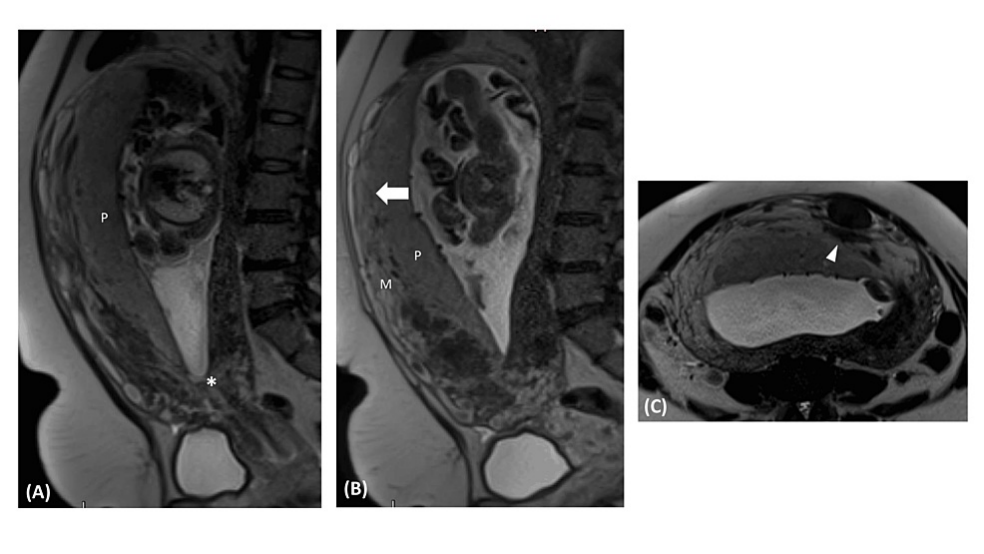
Fetal MRI. (A) Sagittal T2 single-shot fast spin echo (SSFSE) shows anterior placenta (P) in an abnormally low-lying position, distancing 1.4 cm from internal cervical os (*), diagnosing placenta previa. (B) and (C) Sagittal and axial T2 SSFSE reveal additional findings suggestive of placentary invasion of the myometrium (M), such as in definition or thinning of retroplacental T2 dark border (large arrow in B) and dark bands (arrowhead).
